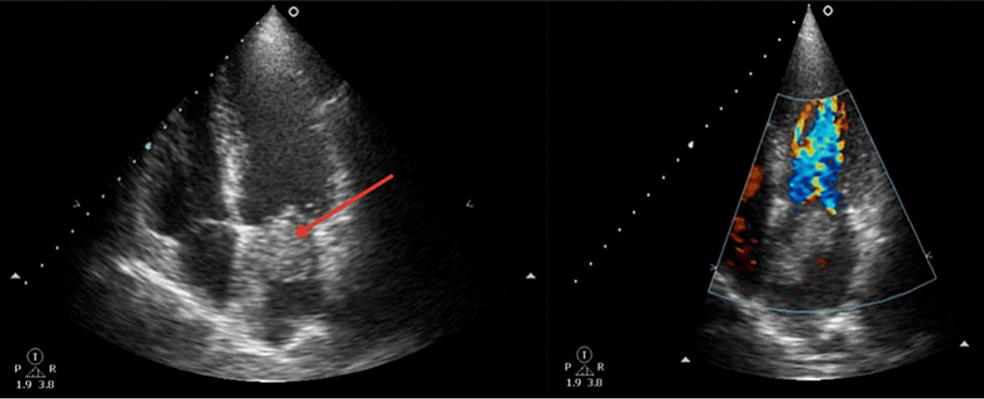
Echocardiogram detects an intracardiac mass in the left atrium
